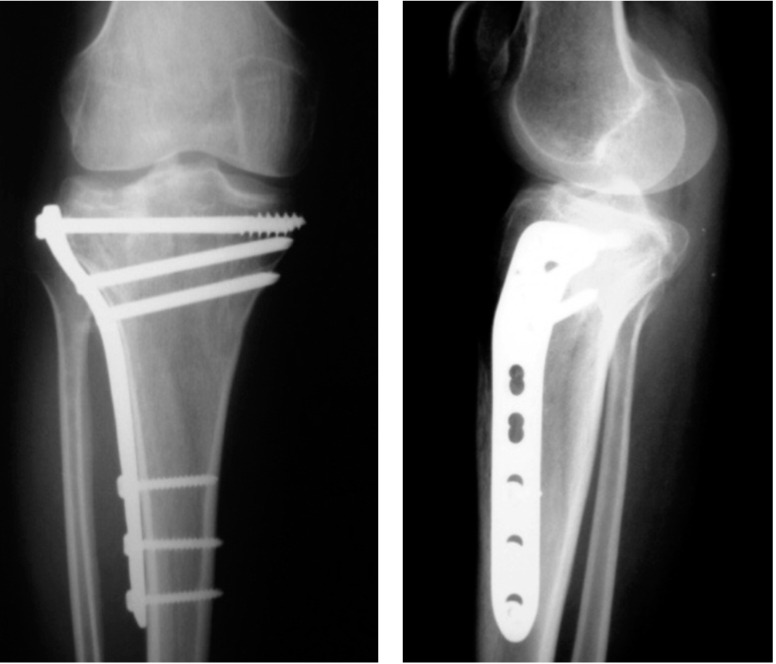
Follow up radiograph at 6 months showing healed fracture and a preserved joint with alignment well maintained.
